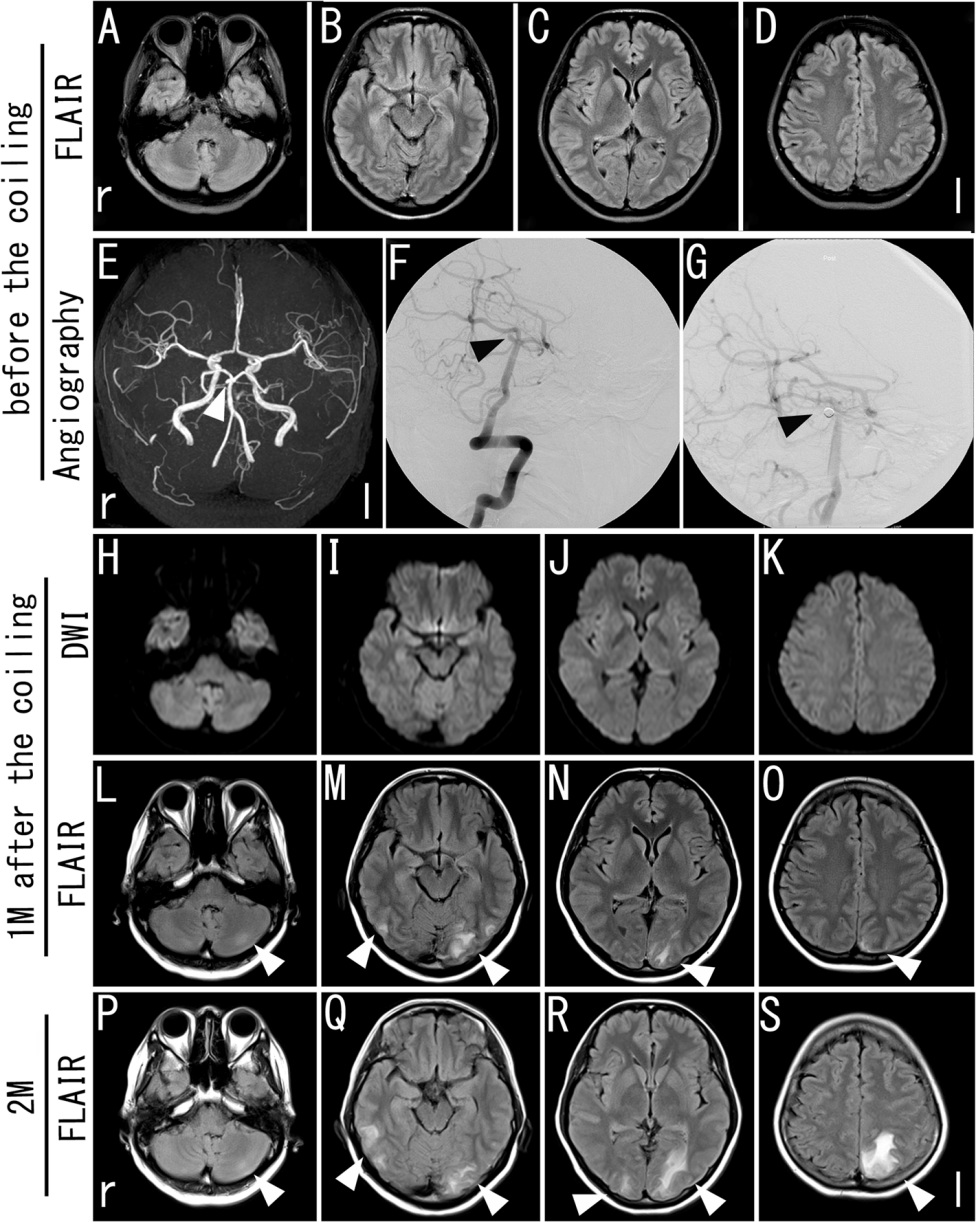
Findings of brain and spinal MRI, and angiography after the first admission for aneurysm coiling and after aneurysm coiling. No lesions were observed with axial slices of a fluid-attenuated inversion recovery (FLAIR) image (A-D). Brain MRA showed a 4 × 4 mm unruptured cerebral aneurysm (CAn) in the basilar artery located at the origin of the right superior cerebellar artery (E, arrowhead). Precoiling posterior angiography showed an unruptured CAn (F) and postcoiling angiography showed the occlusion of CAn and the patency of all vessels (G). After aneurysm coiling, left cerebellar, bilateral occipitotemporal, and left parietoccipital lobe lesions were observed with the axial slices of the FLAIR image (L-O, arrowheads) without a positive signal of the diffusion-weighted image (H-K, arrowheads) at 1 month after coiling, which expanded markedly in size at 2 months after coiling (P-S, arrowheads).
